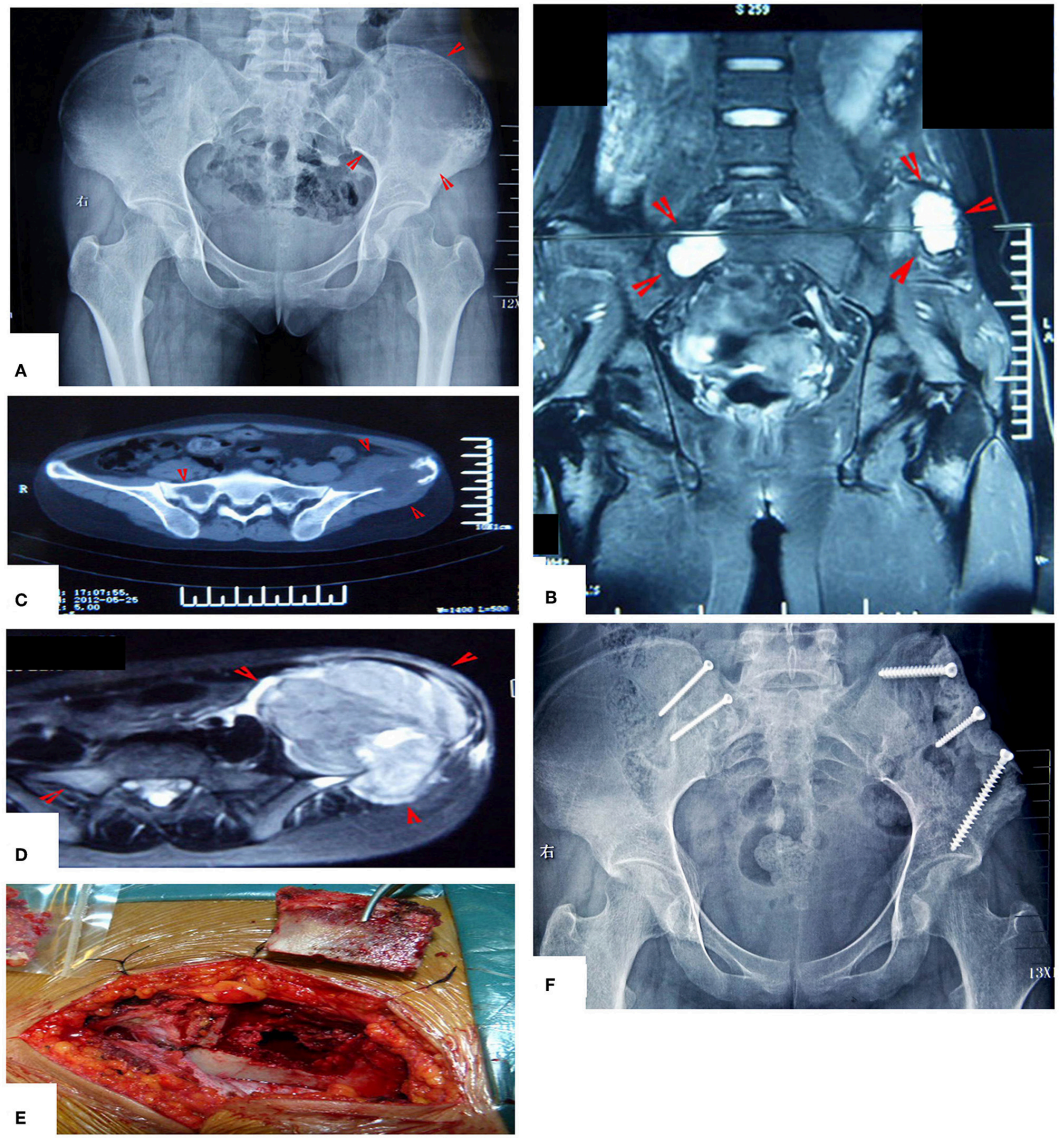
MWA treatment of a patient with bilateral Ewing's sarcoma. (A) X-ray film before operation. (B–D) MRI images show the bilateral lesions. (E) The lesion on the left side was treated as described above. The lesion on the right side was treated through a bone window and the bone bloc was fixed *in situ* by two screws after MWA. (F) X-ray after the operation.
